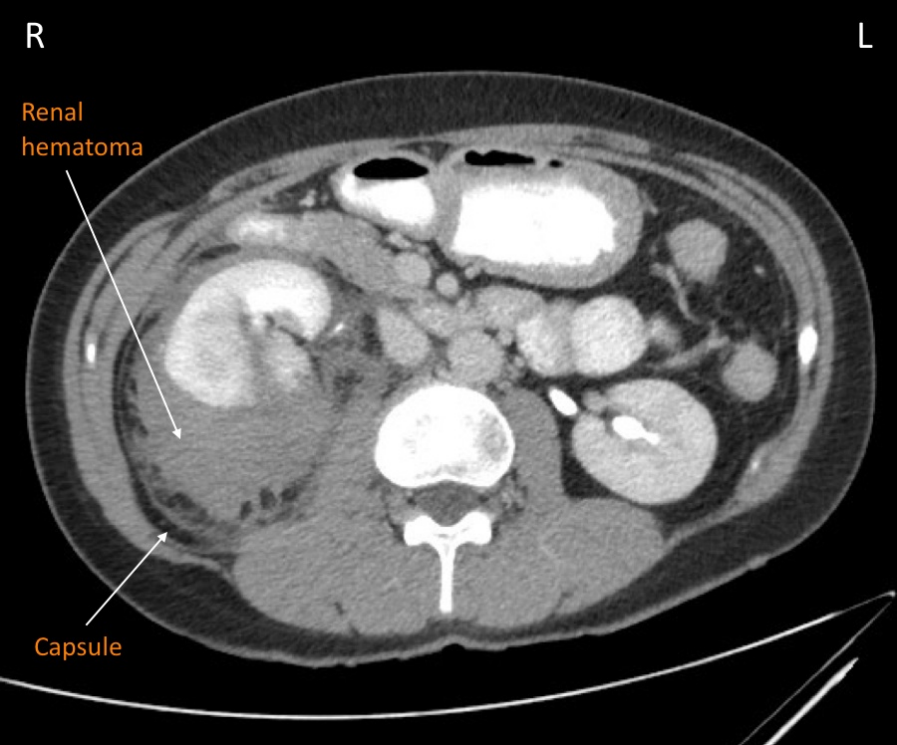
Computed tomography visualization of renal hematoma Coronal view of the abdomen demonstrates Grade IV kidney laceration and large subcapsular hematoma.
